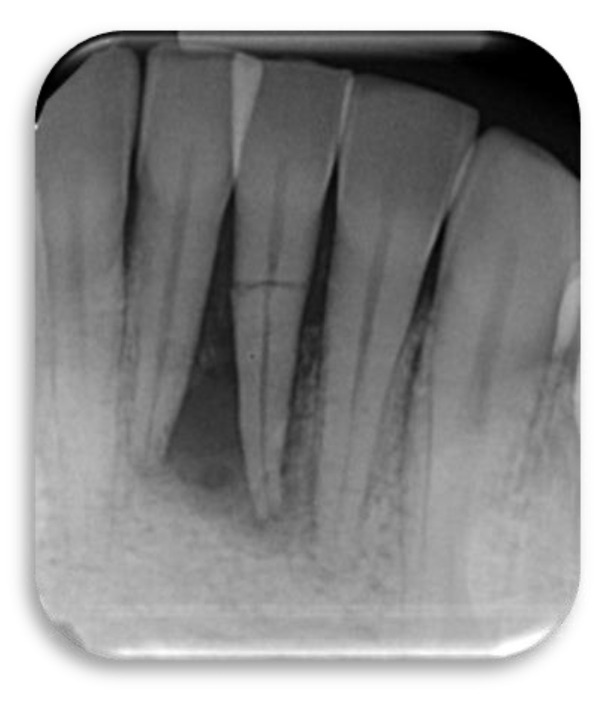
This radiograph shows tooth#24 horizontal fracture and radiolucency in the anterior mandible, as a result of a blow received from the patient playing boxing.
